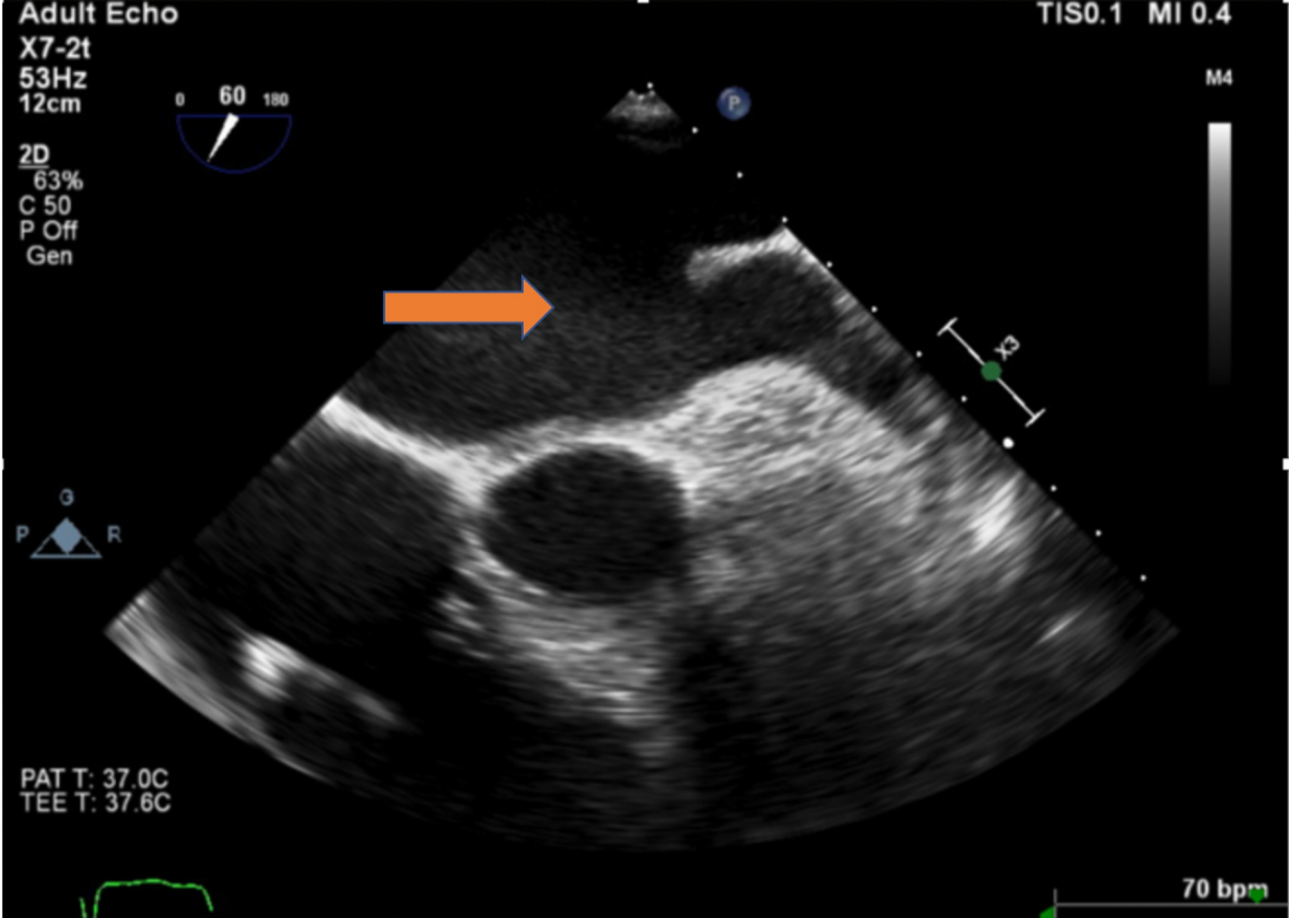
TEE in August 2016 showing left atrial appendage (red arrow) without thrombus. TEE, transesophageal echocardiogram
